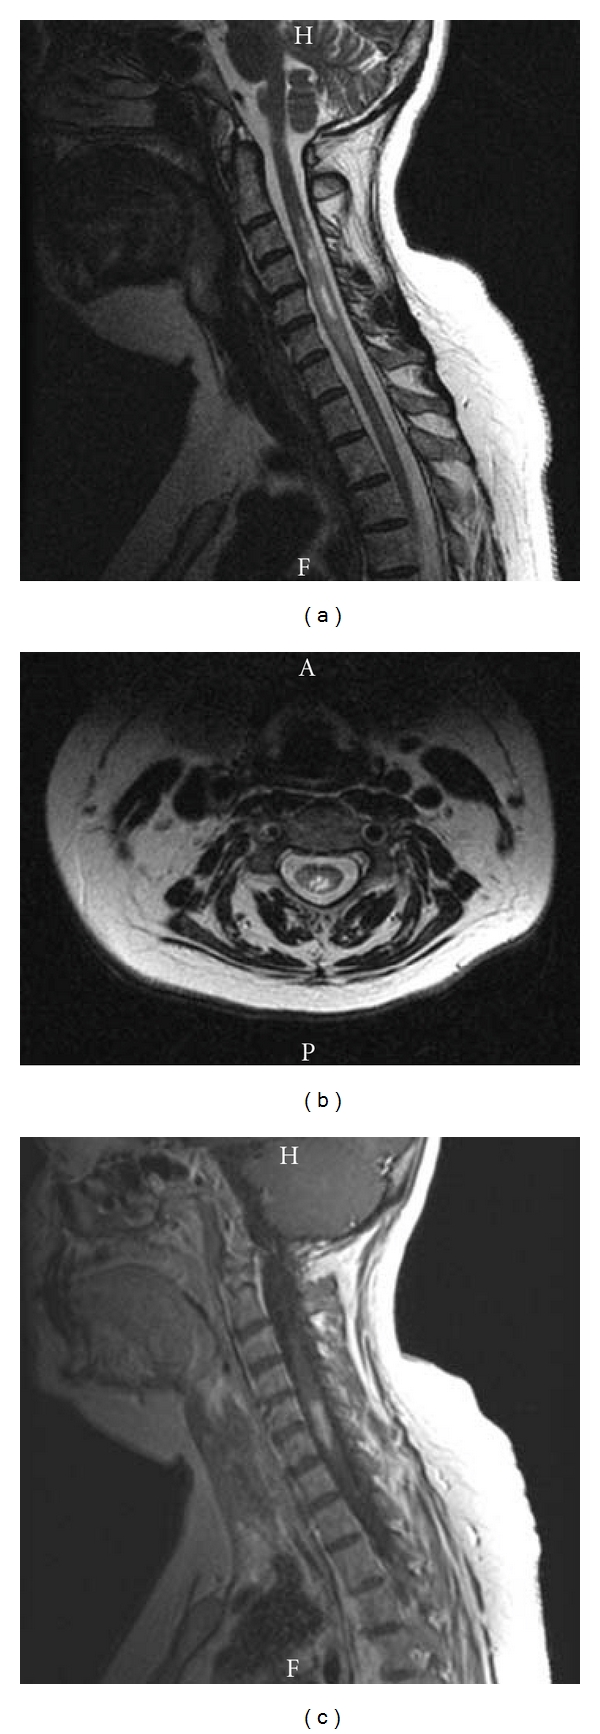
An MRI showing a longitudinally extensive cervical spinal cord lesion in a patient with NMO. (a) T2 weighted sagittal MRI sequence shows T2-hyperintensity extending beyond 3 spinal cord levels. (b) T2-weighted axial sequence shows bilateral T2 hyperintensity in the central/dorsal cord. (c) T1-weighted sagittal sequence with gadolinium contrast shows enhancement in a significant portion of the lesion.
